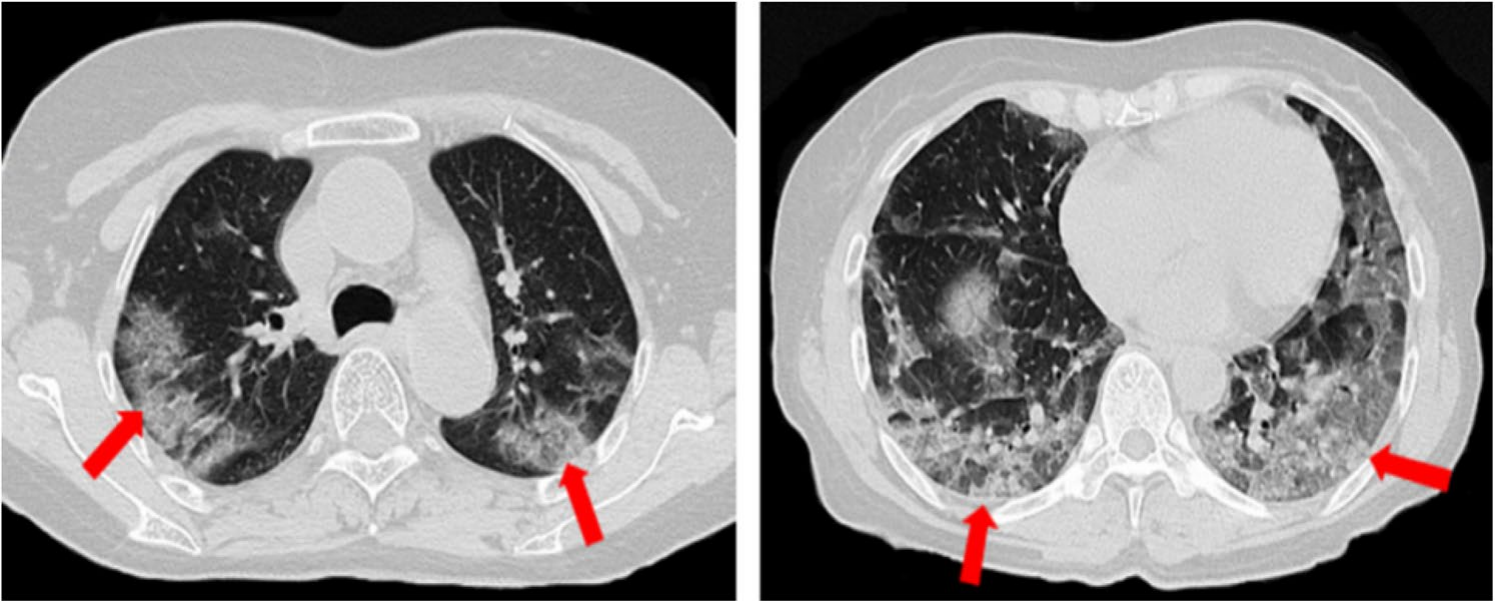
Chest CT feature the crazy-paving pattern. Male, 68 years, confirmed as COVID-19, chest CT images showed crazy-paving pattern (arrow)
